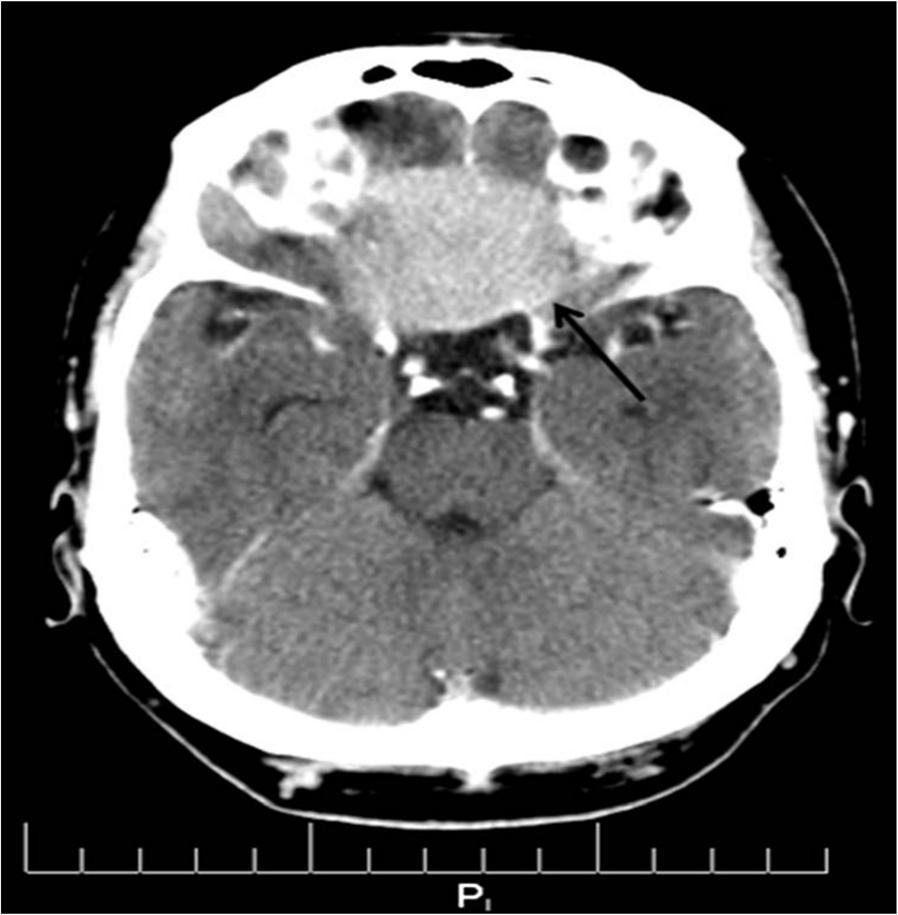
Axial view, CT of the head, soft tissue window reveals a 6x6 cm well marginated meningioma extending
